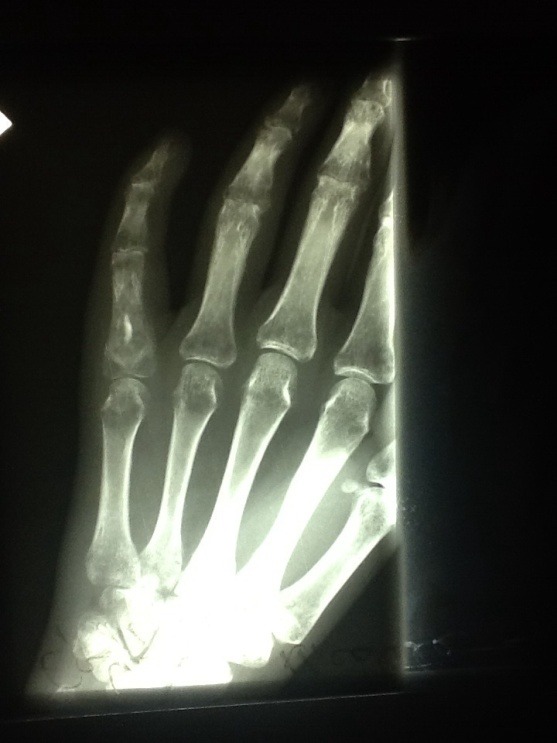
Radiography at 4 weeks postoperatively
